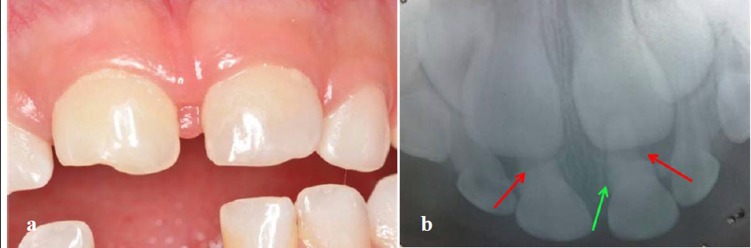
In radiographic examination, external resorption in a “church roof” shape was observed in both teeth, characteristic of teeth that have suffered trauma. Increased periodontal ligament (PDL) space was also observed in tooth 61. Tooth 51 demonstrated calcium degeneration (Figure 1b).
